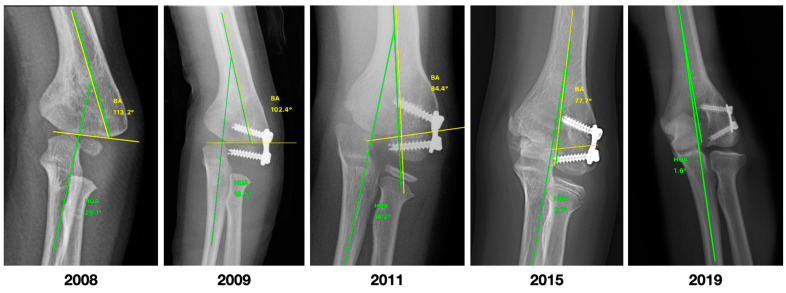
Patient treated with LDHH at the age of 3 years and 5 months, and radiographic images obtained over the following 10 years until the correction of the arm axis. BA, Baumann angle; HUA, humero-ulnar angle.
